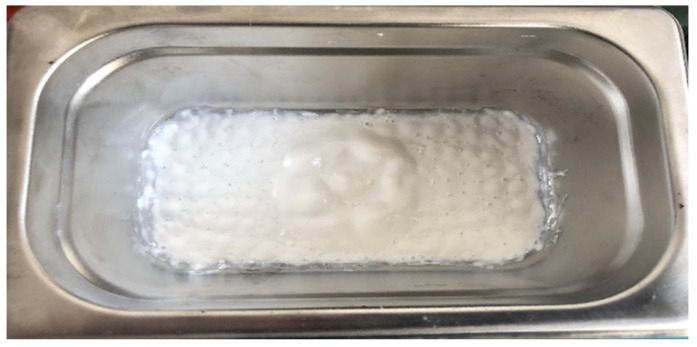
Ultrasonic vibration of titanium dioxide.
